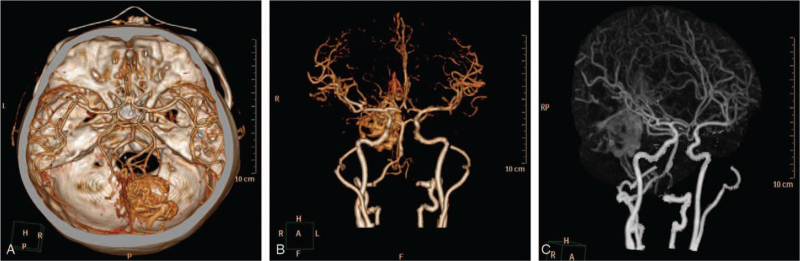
(A–C) Preoperative CTA images showing very rich vascular supply at the tumor bed. CTA = Computed tomography angiography.
